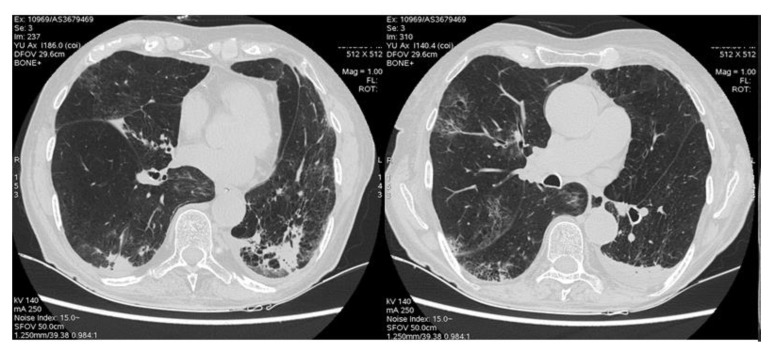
CT features of one patient diagnosed with nivolumab-related drug toxicity in the Siena Centre. Bilateral peripheral parenchymal consolidations involved lower lobes, associated with patchy GGO in the middle lobe and lower lobes and left pleural effusion. The patient was hospitalized due to dyspnea and chest pain.
